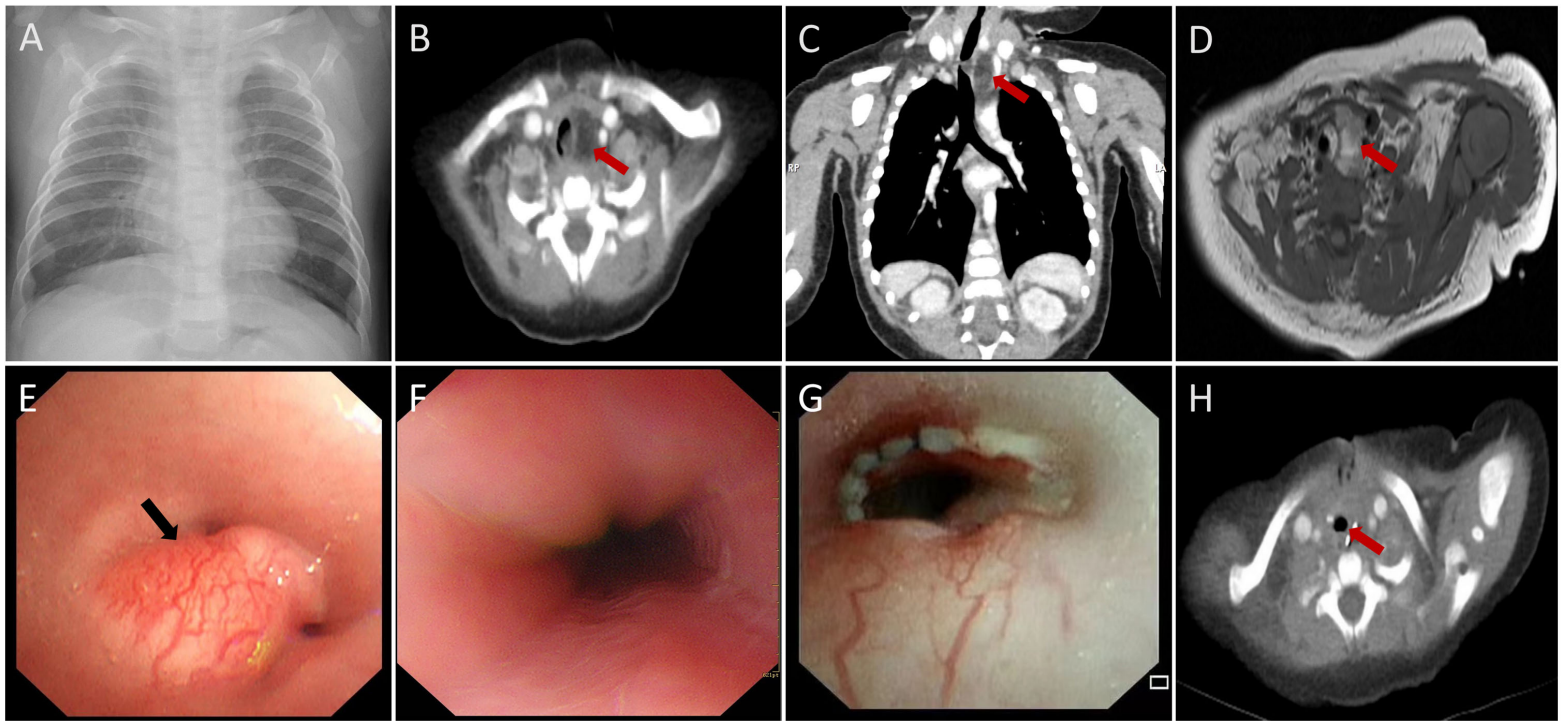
Imaging of mediastinal and endotracheal mass (A) Chest radiography demonstrated no obvious mediastinal mass. (B-D) CT and MRI revealed a mediastinal and endotracheal fatty mass measuring 1.4×1.2×2.3 cm. The trachea was markedly compressed, and the mass protruded into the tracheal lumen, resulting in significant tracheal stenosis (red arrows). (E) Bronchoscopic examination revealed a smooth-surfaced obstructing mass in the upper segments of the tracheal lumen, surrounded by prominent blood vessels (black arrow). (F) Esophageal examination showed no abnormalities. (G, H) MRI scans taken two months postoperatively and postoperative bronchoscopy revealed no recurrence of the mass (red arrows).
